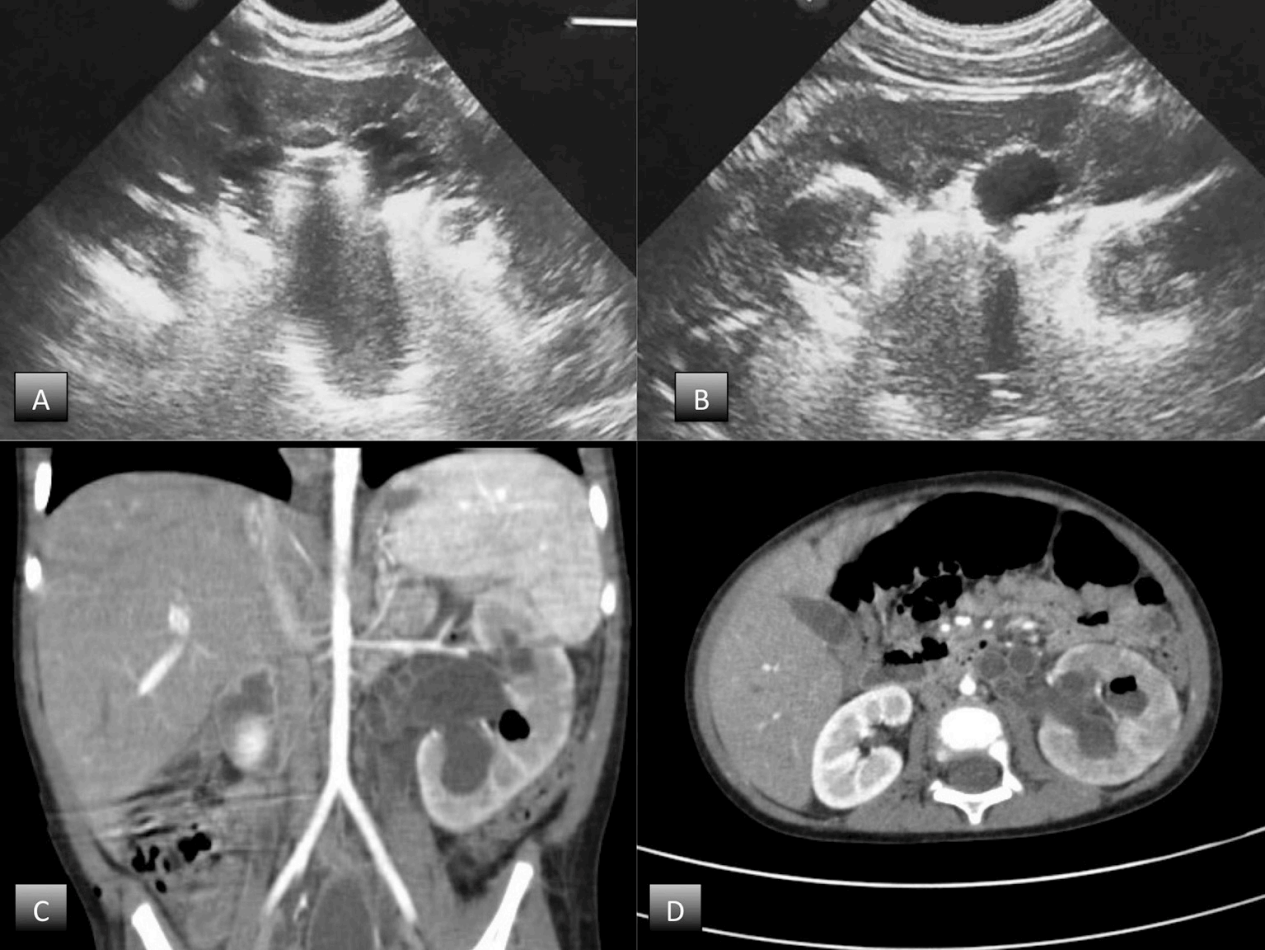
Radiological findings. (A,B) abdominal ultrasound; (C,D) abdominal CT scan with contrast.
